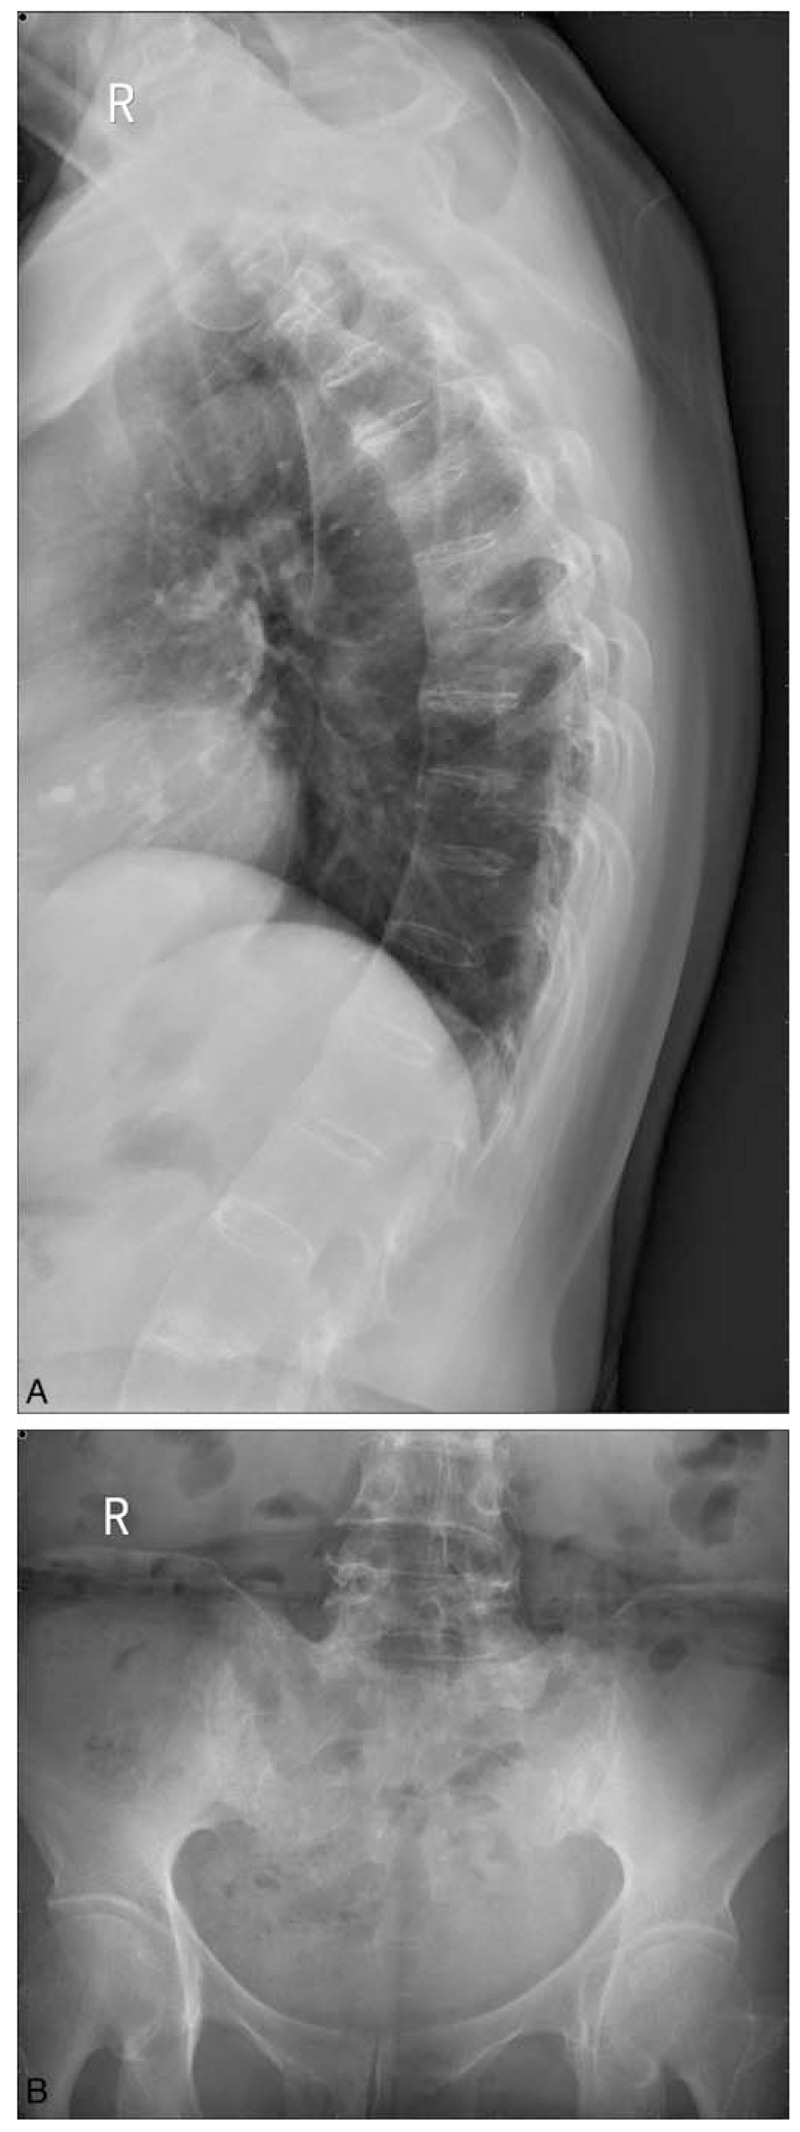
(A) Chest X-ray showed that the thoracic vertebrae underwent a bamboo-like change, but there was no narrowing of the intervertebral space. (B) Pelvic X-ray showed an increased density of bilateral sacroiliac joints and uneven joint gaps.
